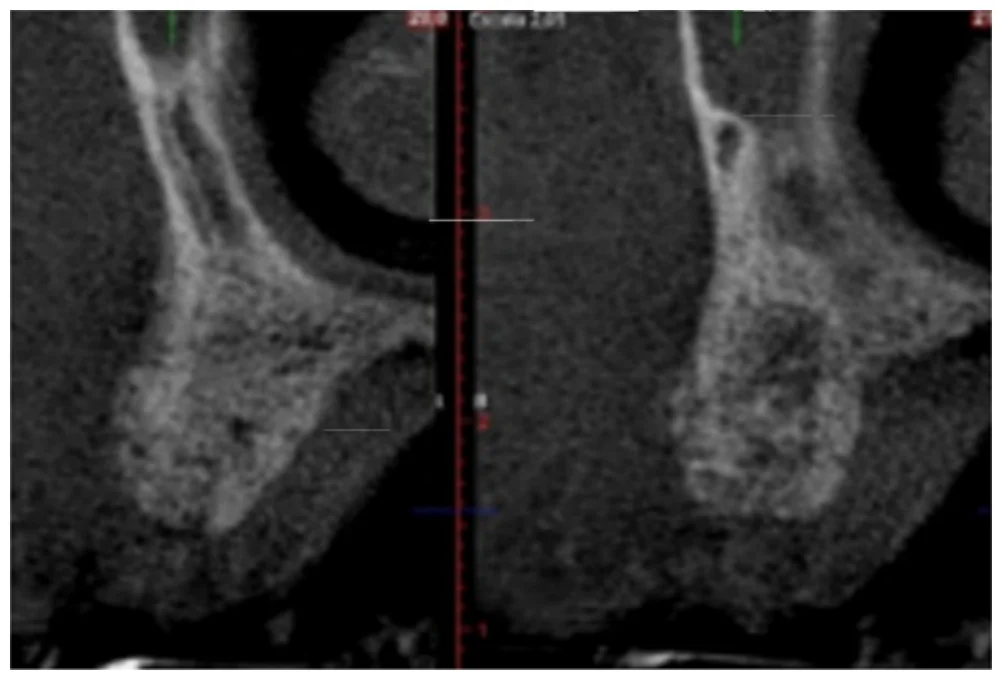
Four-month postoperative CBCT cross-sectional image demonstrating regeneration of ridge width and maturation of grafted bone.
